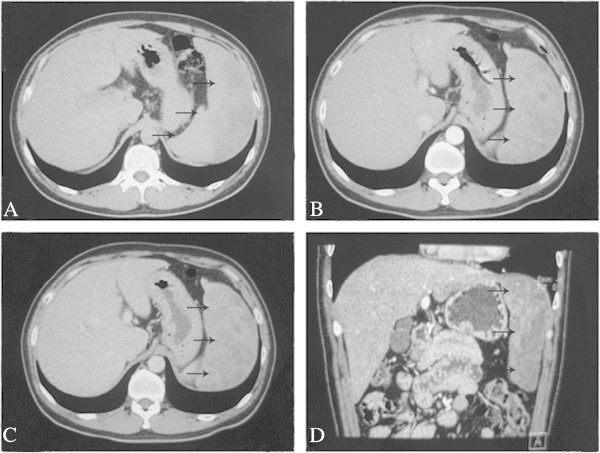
Contrast-enhanced CT scan shows heterogeneous enhancement of the giant cystic lesion. A) the plain CT scan, B) the arterial phase of CT, C) the portal venous phase of CT, D) CT scan in the sagittal plane. Arrows indicate the hepatic lesion.
